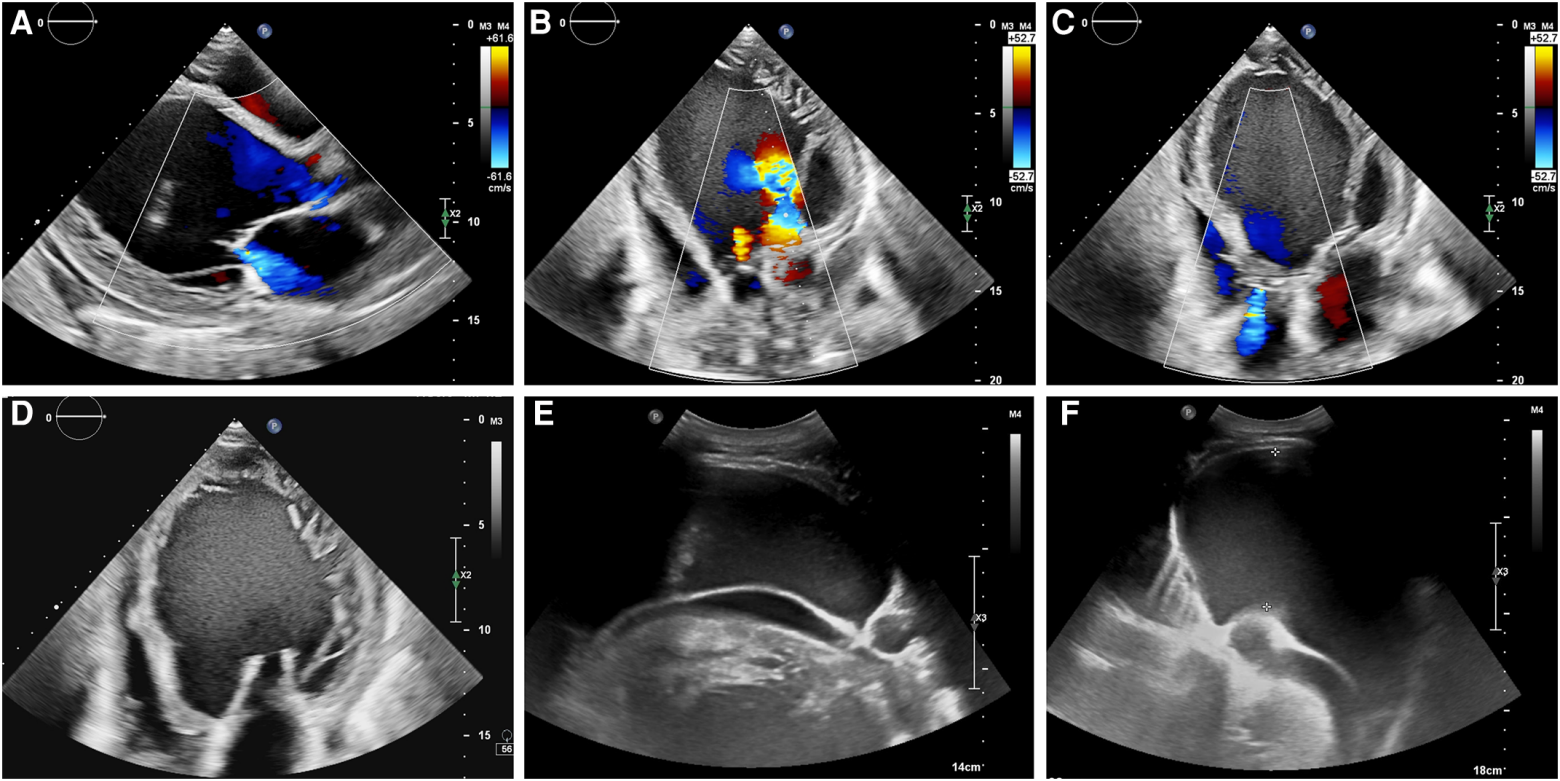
(A–D) The echocardiogram showed left ventricular enlargement of 9.9 cm, and left ventricular systolic and diastolic function were decreased, with a small amount of pericardial effusion. (E,F) Abdominal ultrasound showed the presence of abdominal fluid accumulation, also known as ascites.
